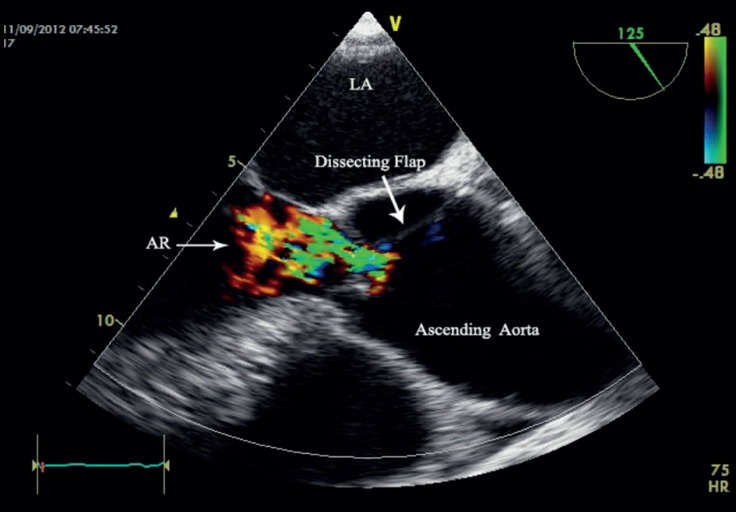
Transesophageal echocardiography in the mid-esophageal view, showing severe aortic regurgitation (AR) and a dilated ascending aorta
